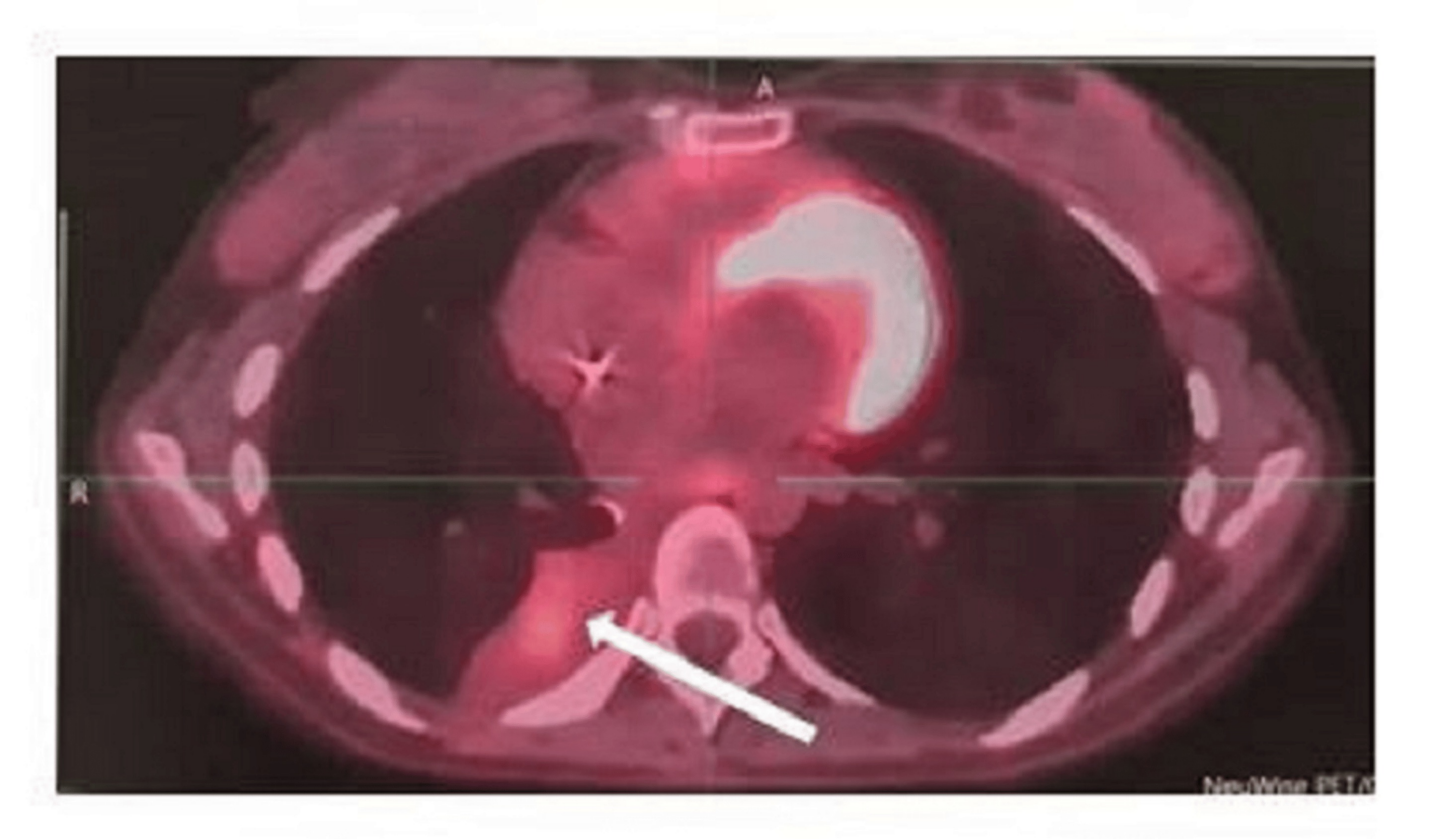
PET/CT scan after the end of treatment revealing a favorable metabolic response and regression of pulmonary metabolic activity (white arrow).
